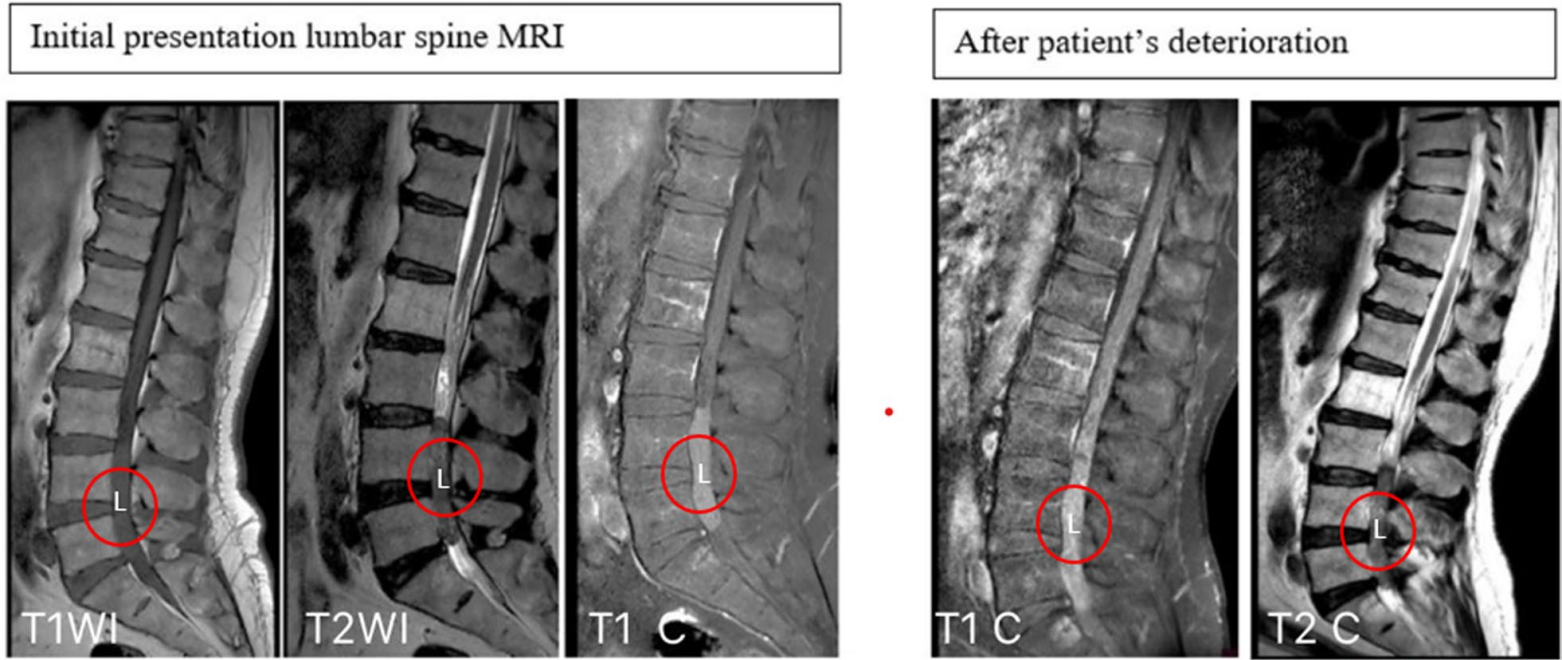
Sagittal lumber MRI images at the initial presentation and after the deterioration of the patient’s status. At the initial presentation, T1 and T2 noncontrasted sequence showing intermediate T1, low T2 signal-intensity intradural/ extramedullary lesion extending from the lower aspect of L3 to L5–S1 disc level, while sagittal T1 contrasted image showing diffuse homogenous enhancement of the lesion. After the deterioration of patient’s symptoms, sagittal T1- and T2-contrasted images showing change in intensity in T2 and change in enhancement with contrast to a heterogenous pattern, suggestive of intratumoral hemorrhage. L: lesion; T1WI: T1 MRI image without contrast; T2WI: T2 MRI image without contrast; T1 C: T1 MRI image with contrast; T2 C: T2 MRI image with contrast (red circle indicates the letter L)
